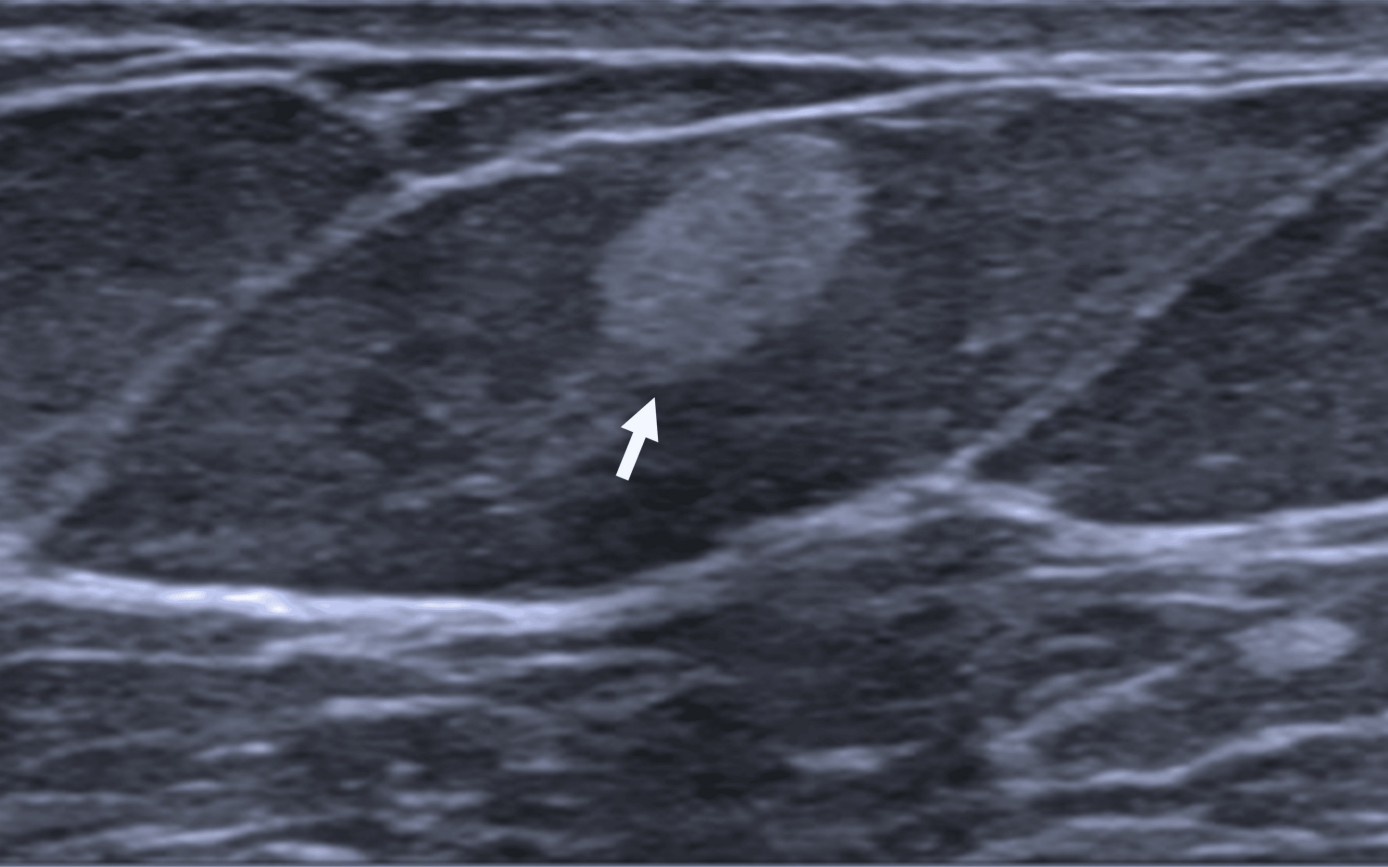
Ultrasound image showing the mass (white arrow) as a 0.7 × 0.7 × 0.4 cm hyperechoic lesion.
